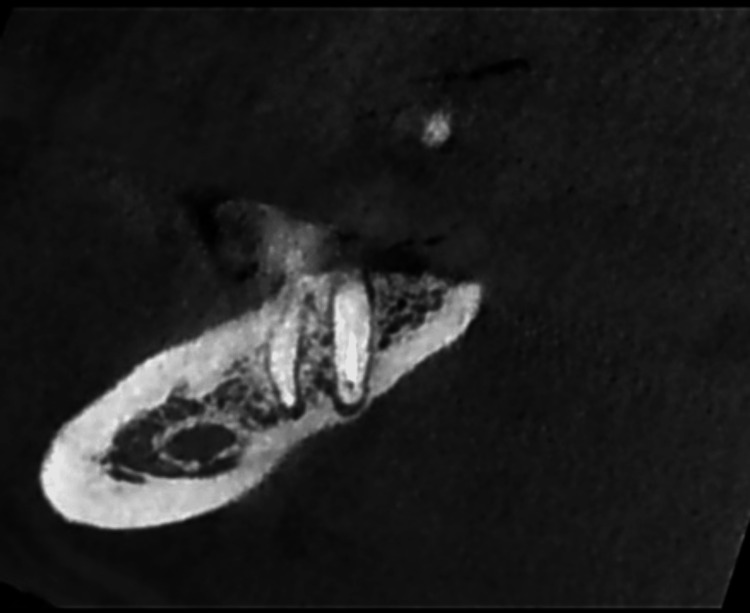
CBCT image of tooth 47 showing the radiolucency on both roots in sagittal section. CBCT, cone beam computed tomography.
